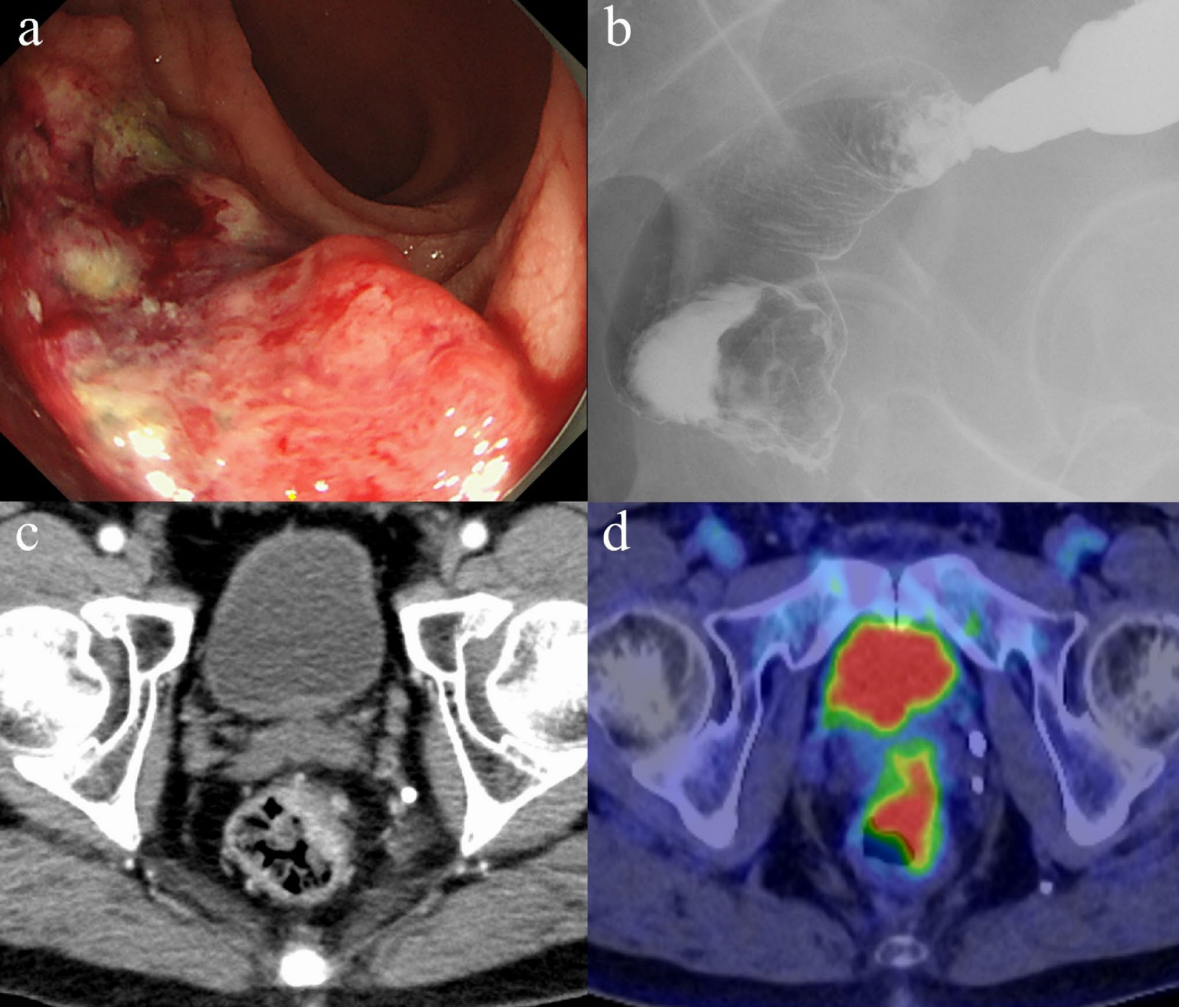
Results of the examination before the surgical treatment. a: Colonoscopy revealed a 25 × 20 mm tumor was located 5 cm above the anal verge on the anterior wall of the lower rectum. b: Gastrografin enema showing deformity in the anterior wall of the lower rectum. c: Contrast-enhanced CT tomography showing irregular wall thickening from the anterior to the left wall of the lower rectum (approximately half the circumference). d: Positron emission tomography-CT showed abnormal accumulation in the left wall of the anterior wall of the lower rectum
